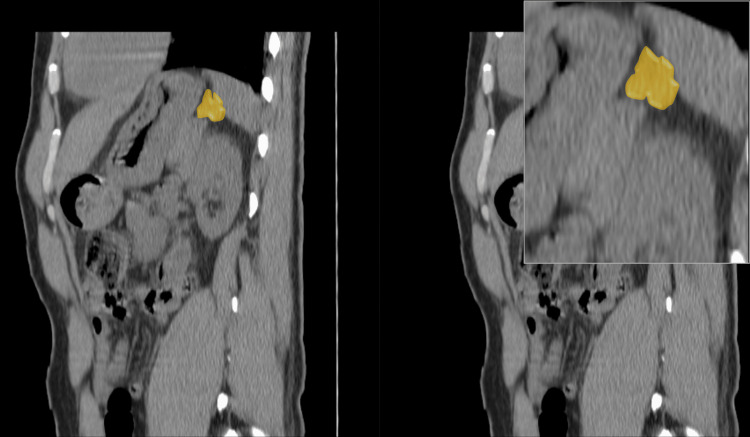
Non-contrast computed tomography scan of the abdomen and pelvis (coronal view). Severe left adrenal thickening (yellow).
